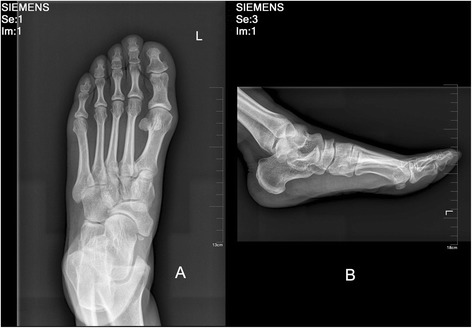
a Anteroposterior and b lateral radiograph of the foot indicate a Mueller-Weiss disease preoperatively
